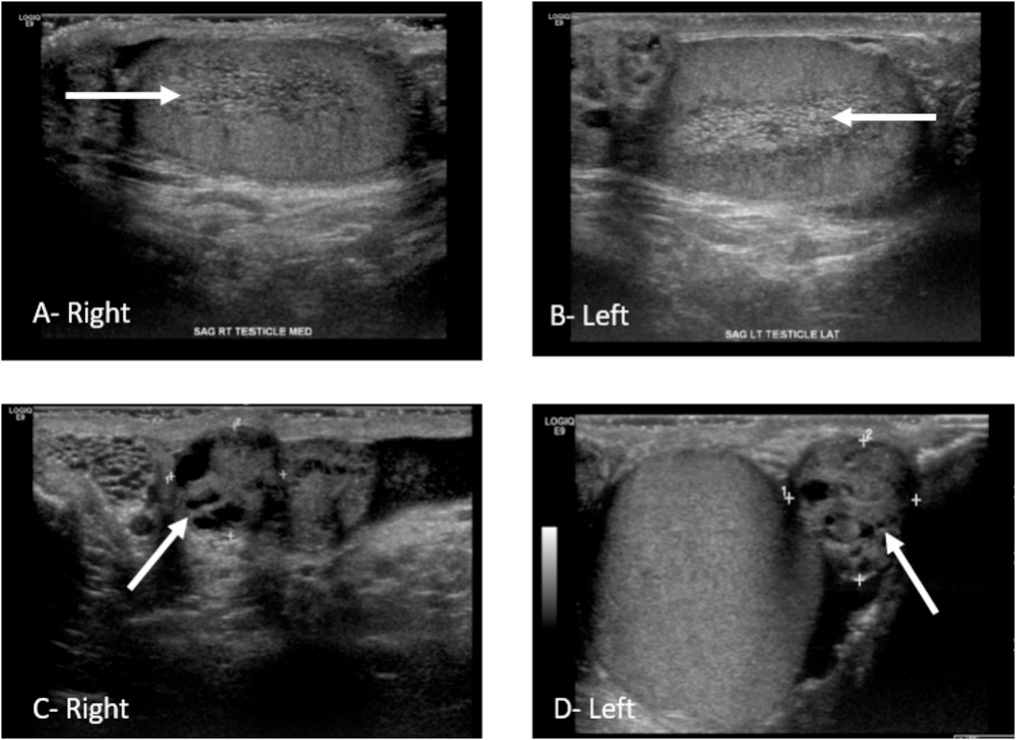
Ultrasound Images. Right and Left Testicles noted dilated rete testes (Arrows-Images A, B); Bilateral epididymal lesions with mostly solid components and small cystic areas consistent with cystadenomas (Arrows-Images C, D).
